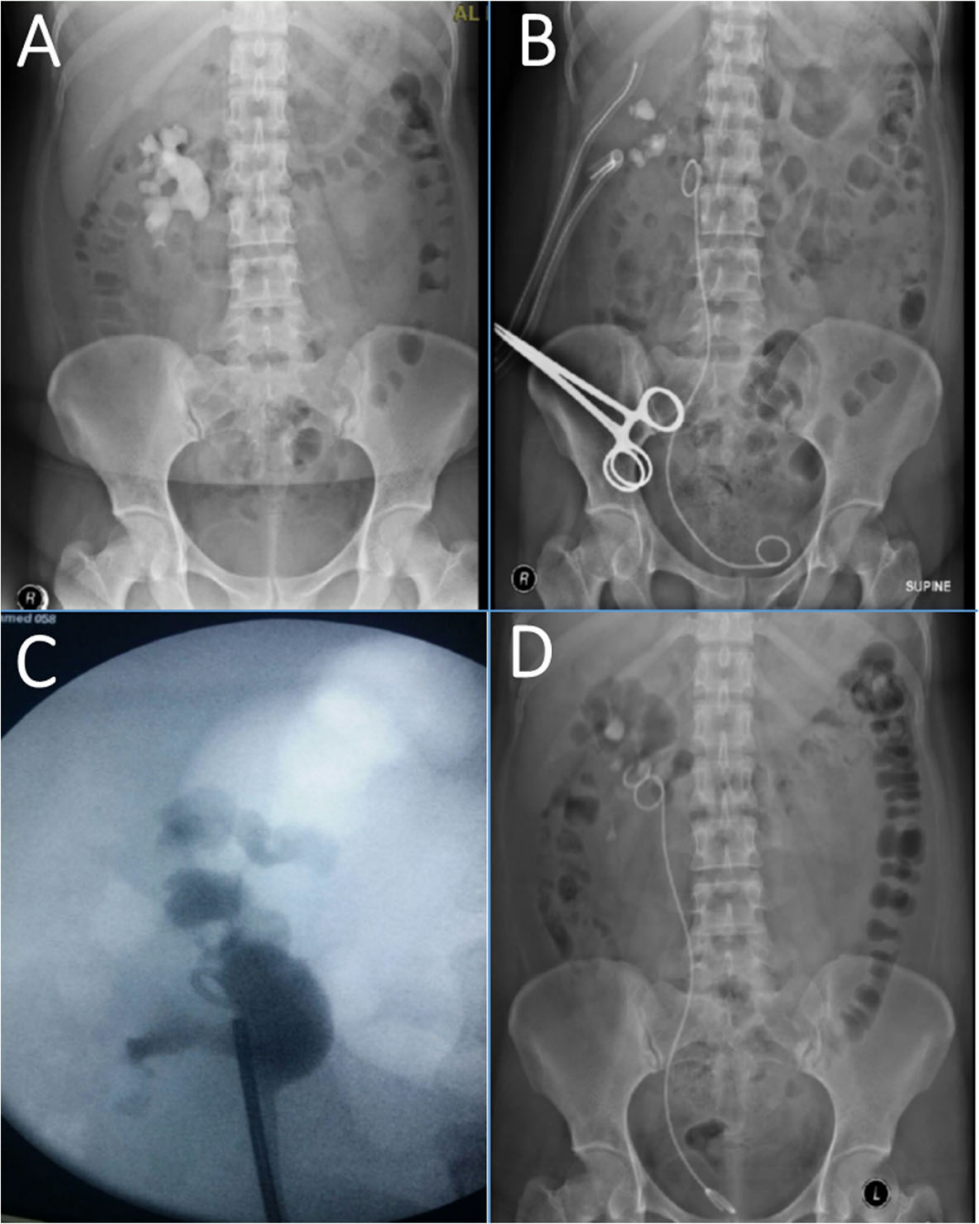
Imaging of the second case. a, Pre-operative plain x-ray with full staghorn calculus; b, Immediate post-operative plain x-ray showing the two nephrostomy drainage tubes clamped and the JJ stent in position with the remnant stone fragments; c, Retrograde pyelography showing the loop of the JJ stent outside the collecting system; d, Plain x-ray showing isolated upper coil of the embedded stent following transection of the stent at the junction of the coil with the stem using rigid ureteroscopy. Another JJ stent was placed during the procedure
